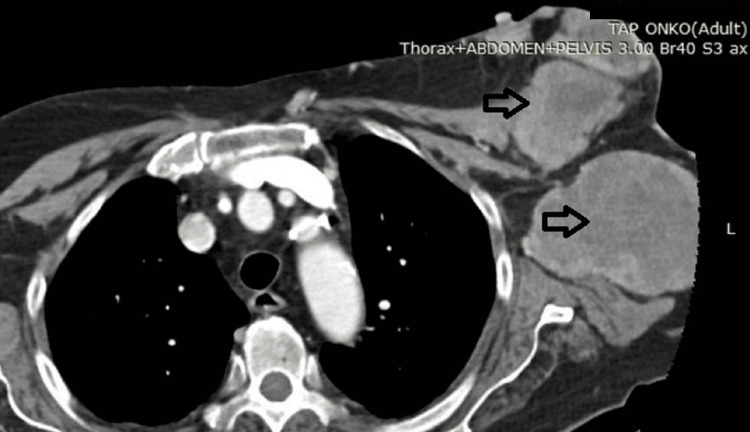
The preoperative computed tomography with the lesions marked by arrows
